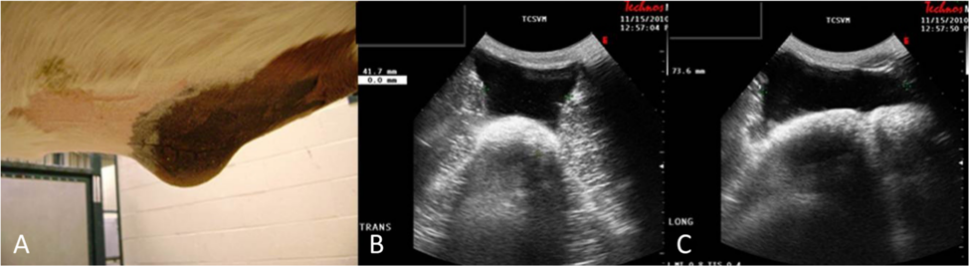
Preoperative gross (A) and ultrasonographic appearance of the ventral midline hernia using a 5 MHz convex abdominal ultrasound probe in transverse (B) and longitudinal (C) planes revealing peritoneal fluid filling the hernia sac. Measurement markings on right side of the ultrasound images indicate 0.5-cm intervals. E indicates the cranial and lateral margins of the defect.
